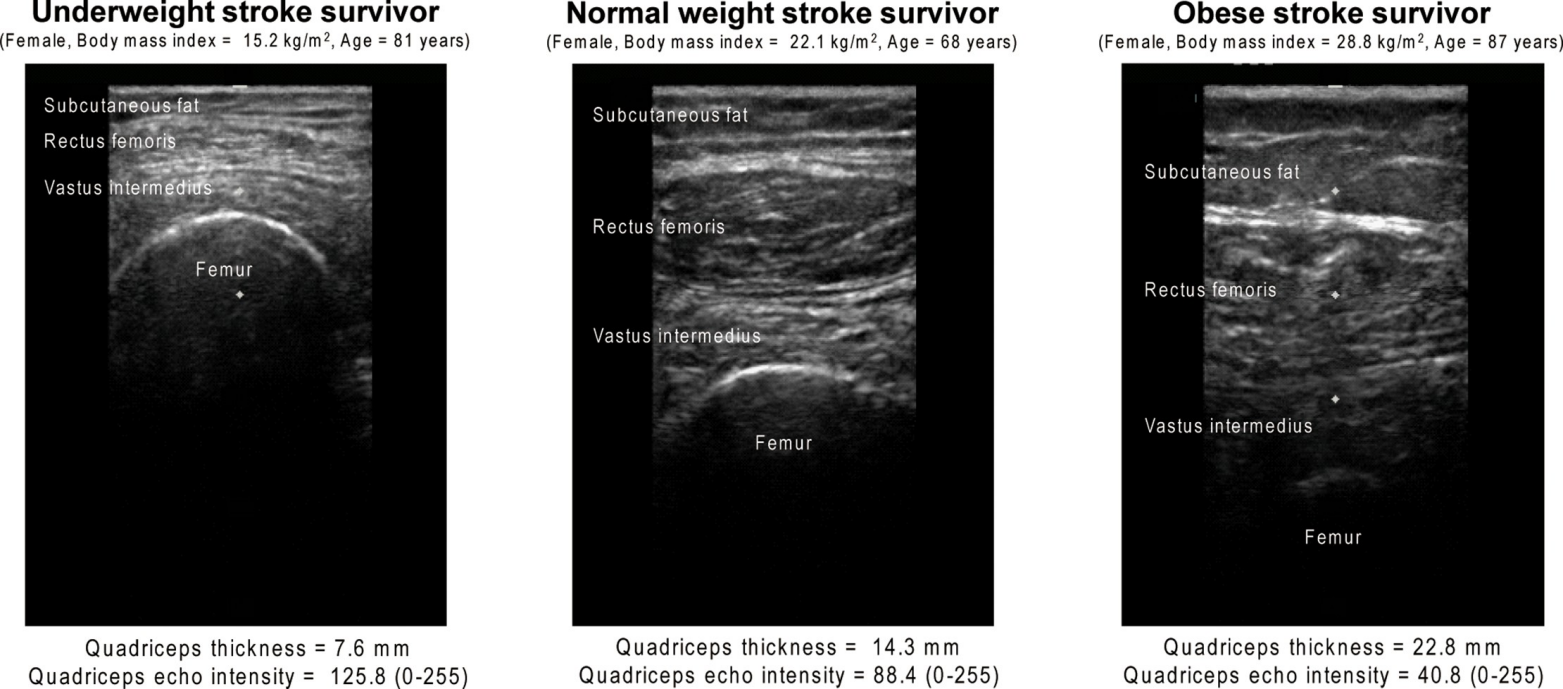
Typical ultrasound images on the paretic side of patients with different body mass indexes.
